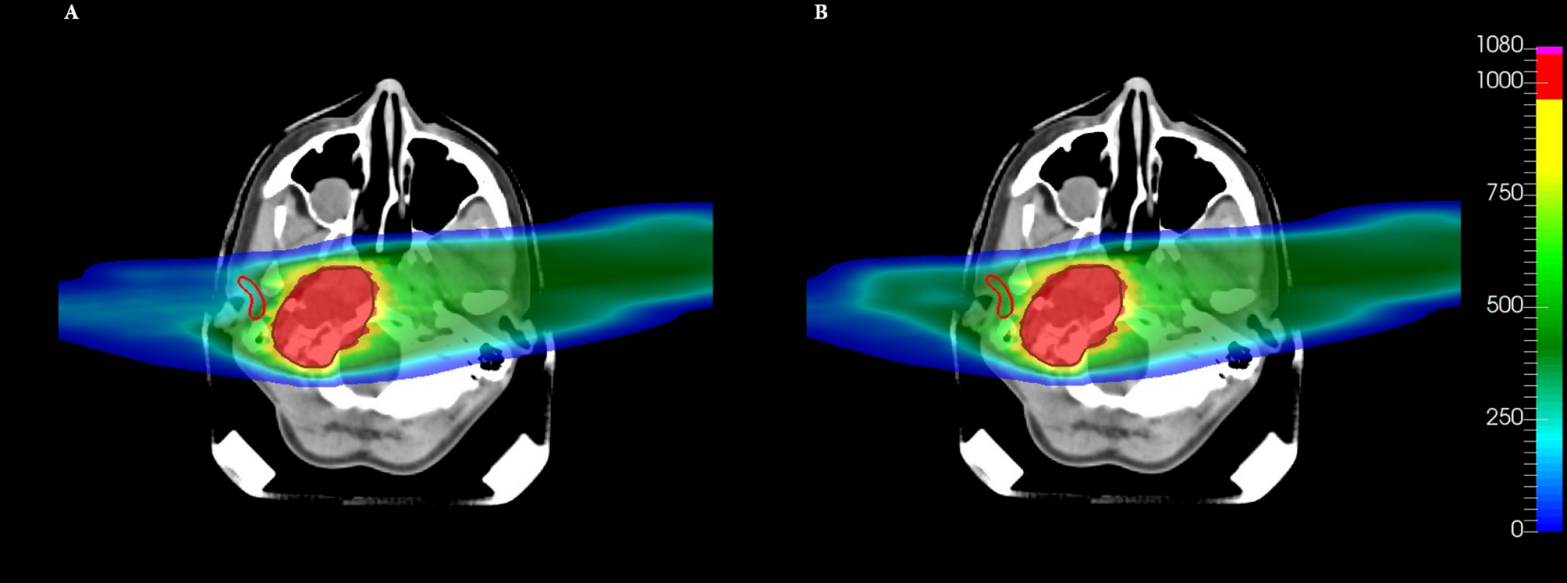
Comparison of dose distributions on a CT slice for different volume effect parameters *a* of the parotid. Cost function parameters: (A)
